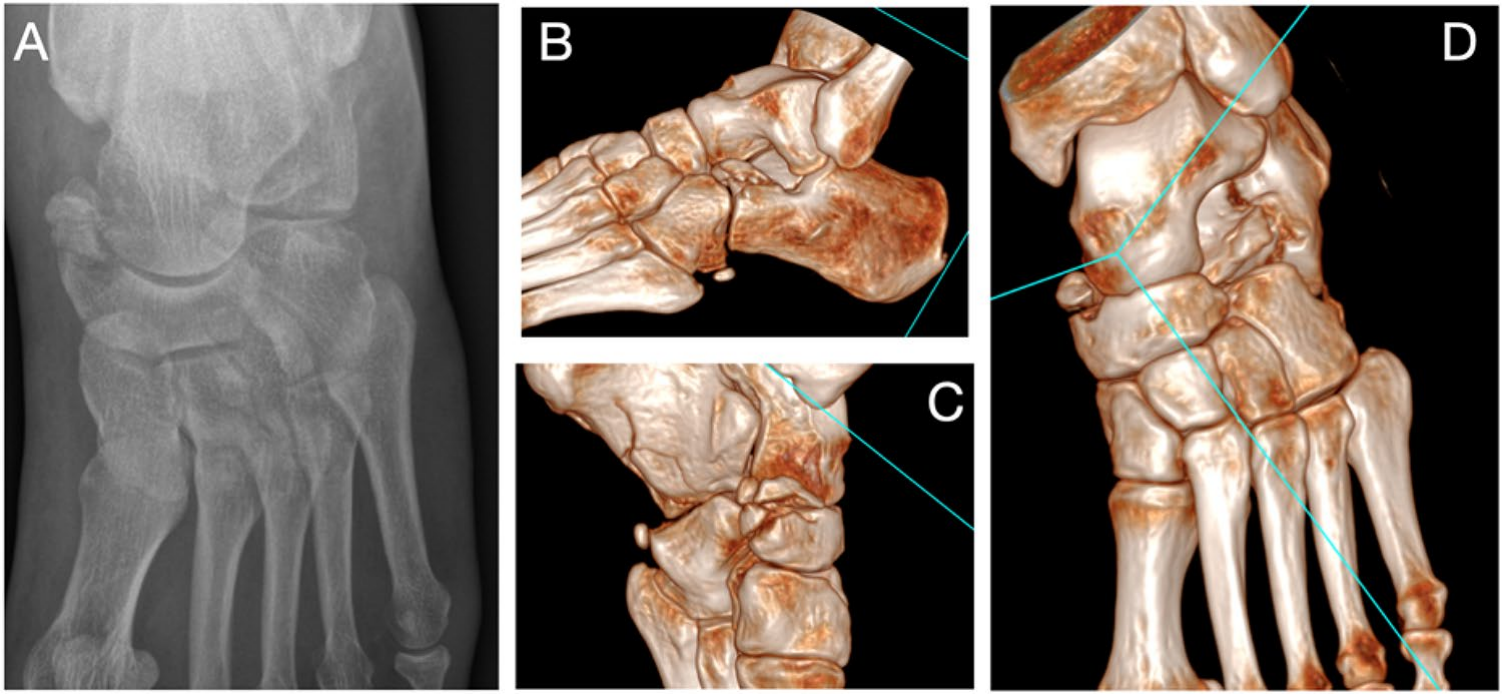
Chopart fracture without dislocation example. Anteroposterior radiograph (A) and 3D Surface rendering CT (B, C and D) of a Lateral compression (Anterior process calcaneum) Chopart joint injury and medial distraction injury with tibialis posterior and spring ligament avulsion from the navicular
